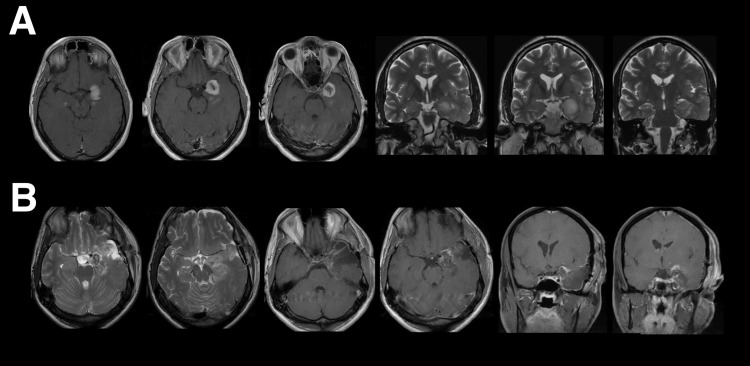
Preoperative and postoperative neuroimaging Preoperative (A) and postoperative (B) axial and coronal gadolinium-enhanced T1 and T2-weighted MR images demonstrating a left hippocampal glioma and the effects of a subsequent total resection.
